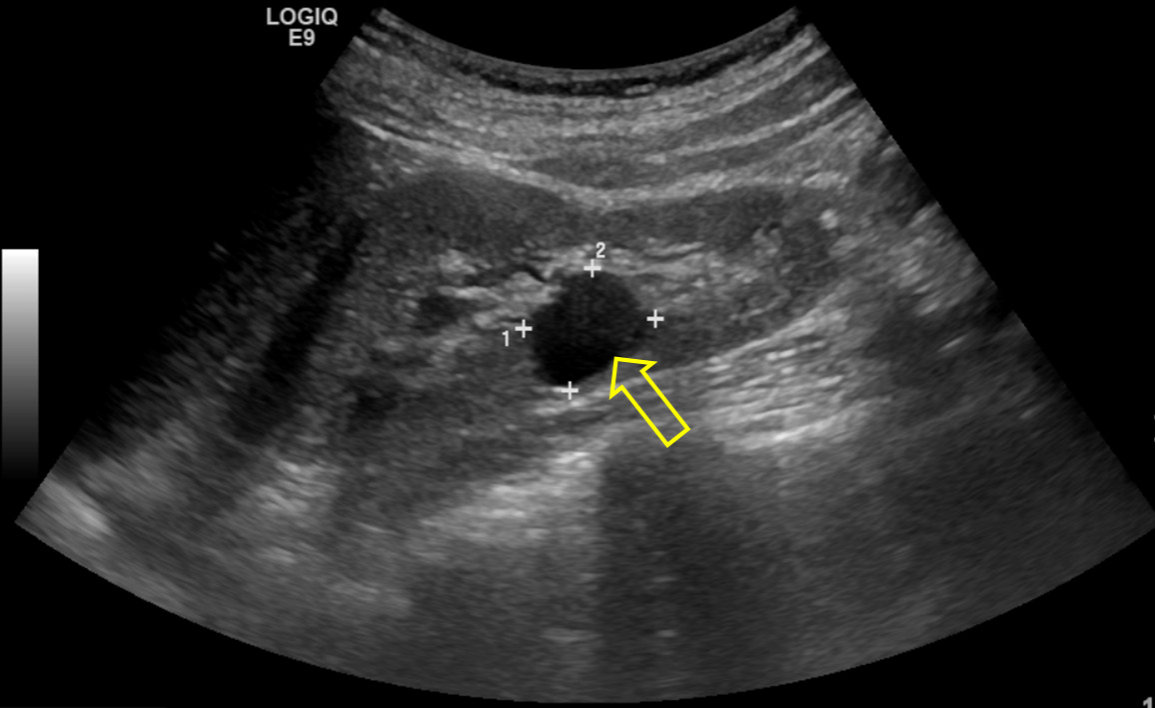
Renal ultrasound showing a renal cyst (arrow).
